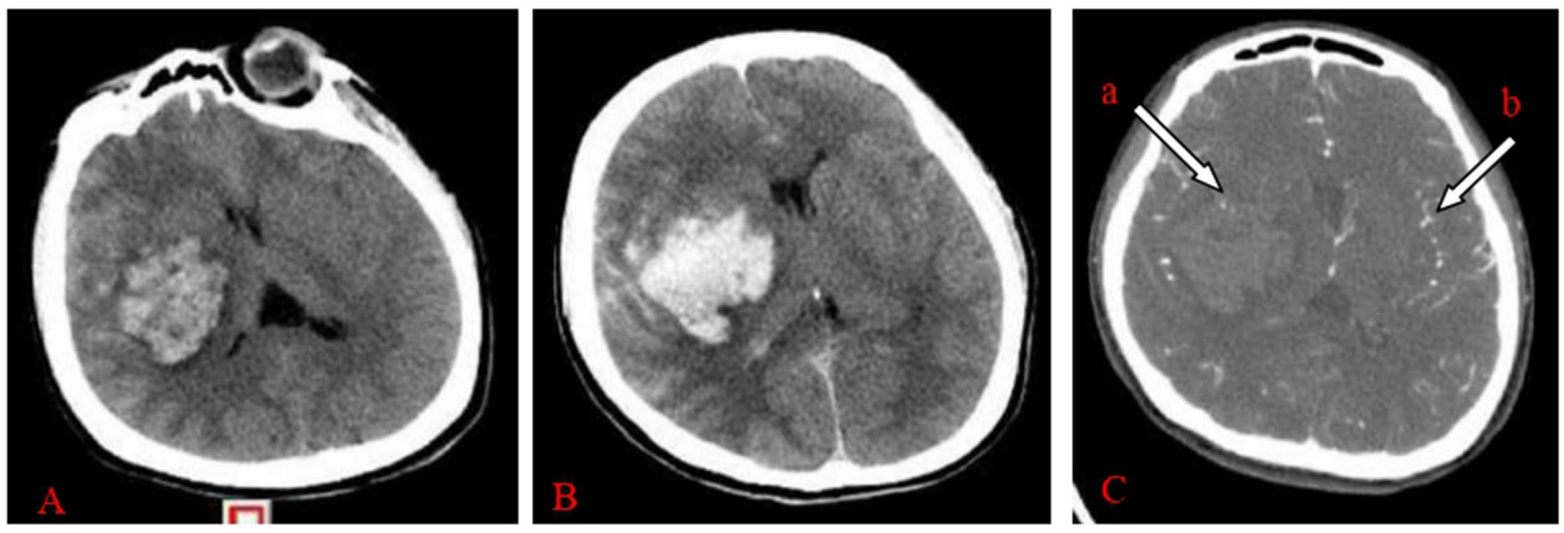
(A–C) Female, 47 years old, experienced a sudden headache accompanied by left leg paralysis for 4 h and hemorrhage in the right basal ganglia region. (A) Initial CT imaging of the right basal ganglia region revealed an anomalous hyperdense shadow, next to the internal capsule, indicating compression of the internal capsule. The Remebot software system immediately calculated the hematoma volume to be approximately 50.17 mL. (B) CTA reveals a hematoma including an internal hematoma, with (a) arrowheads denoting punctate hyperdense enhancement, confirming a positive “hematoma internal point sign.” (b) Arrowheads denote point-like and striated hyperintensity increase, signifying a positive “angiographic point sign.” Upon reviewing the CT plain scan after 6 h of onset, the hemorrhage in the right basal ganglia region had risen, with the Remebot software system automatically measuring the hematoma volume at approximately 57.92 mL.
